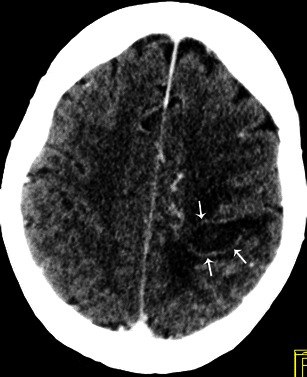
Contrast-enhanced cerebral CT shows a brain abscess as a hypodense, oval shaped lesion with peripheral enhancement (*white arrows*) and surrounding oedema in the left parietal region
